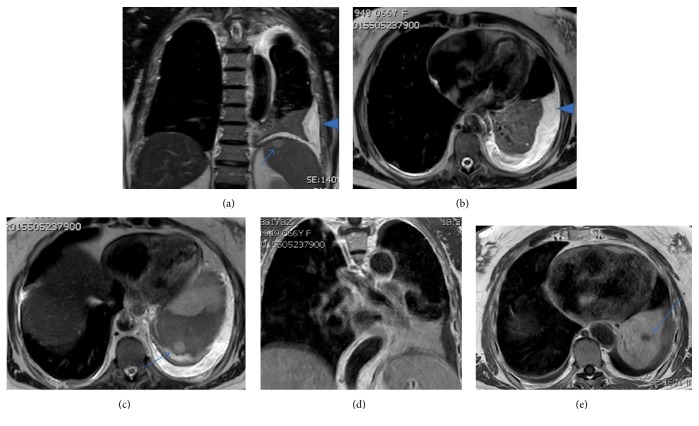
Thoracic MR images. Fluid sensitive sequences (a–c) show the presence of left pleural effusion (arrowhead) and also the nodular lesions in the spleen, which do not correspond to cysts (arrows). T1 weighted images with contrast (d and e) show the pulmonary mass and hypodense zones representing necrotic areas (arrow).
